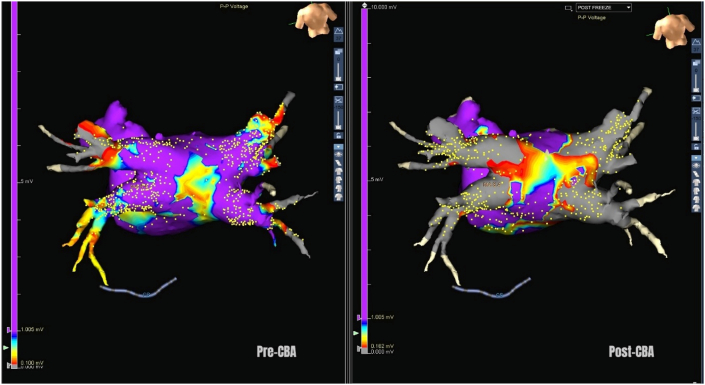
Pre- and post-images of cryoablation PVI created with electroanatomic map-CT fusion imaging.
